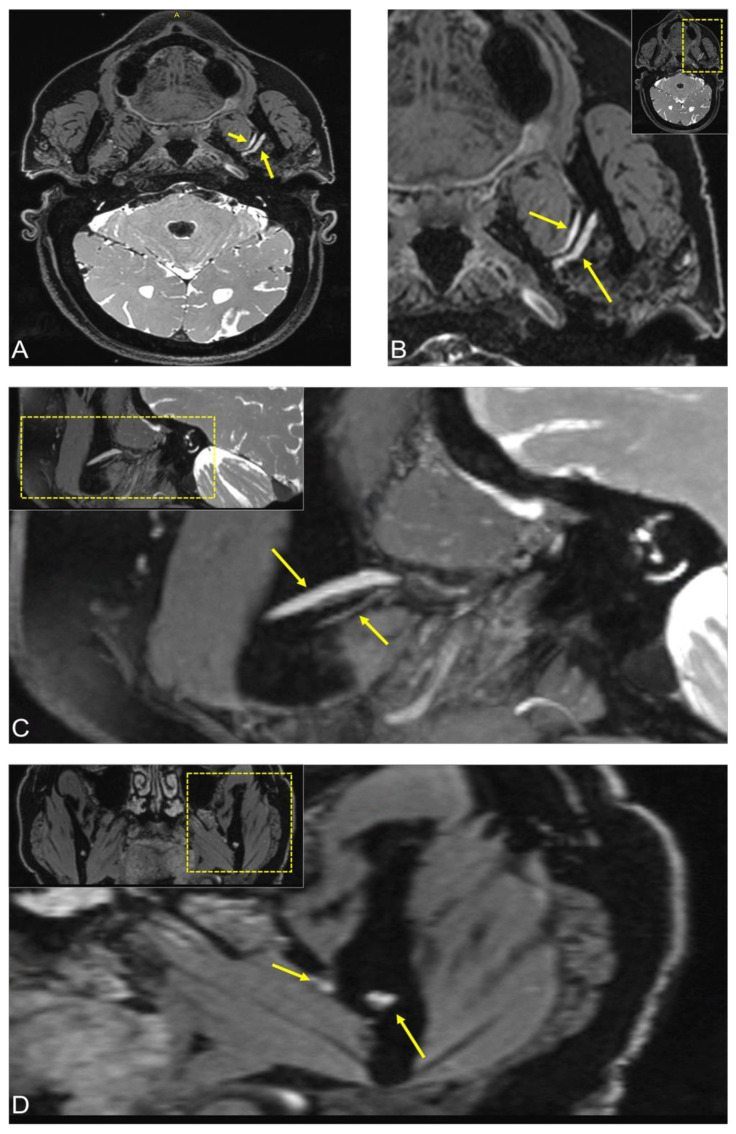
3D Double-Echo Steady State (DESS) MRI of a patient complaining of constant numbness in the left cheek, chin, and mandible, partly with radiation to the ear and temporal area. The DESS protocol showed osseous signal alteration on the left mandible in the third quadrant in the molar region. (A,B) Axial, (C) sagittal, and (D) coronal side-by-side comparison of the image reconstructions showed hyperintense signal alterations of the mandibular nerve from the oval foramen through its branches in the inferior alveolar nerve and lingual nerve to the region of the third molar. In all images, the short arrow shows signal hyperintensities of the lingual nerve at the anatomic point where it begins to pass from lateral to medial, and the longer arrow represents the inferior alveolar nerve before it enters into the mandibular foramen. For orientation, the dotted rectangles in the corner show the enlarged area.
